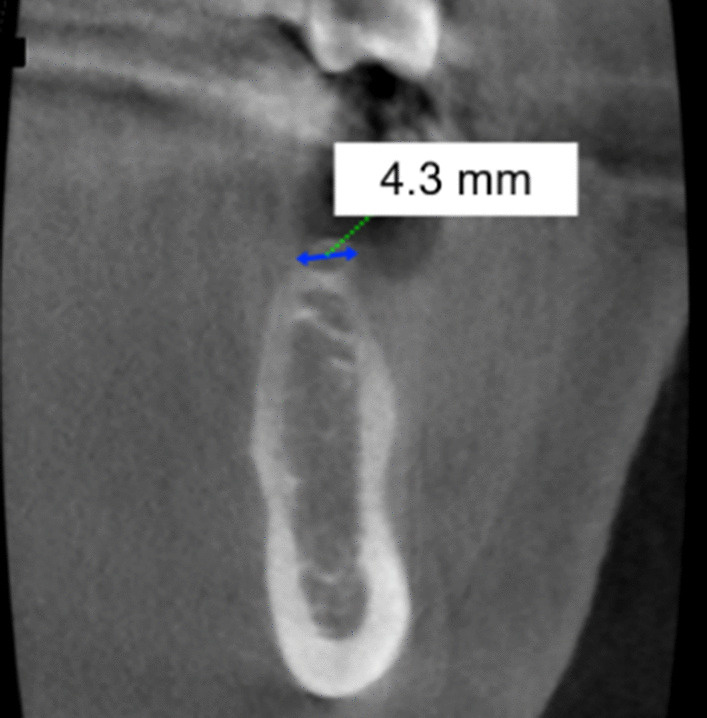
Cross sections from the CBCT of region 36 for case vignette 1. The alveolar ridge is atrophied in the crestal region
